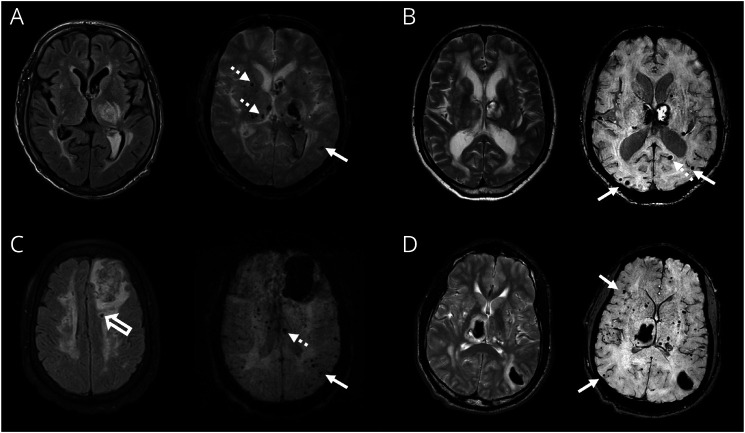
MRI Examples of Patients With Chronic Kidney Disease and Mixed Location Cerebral Small Vessel Disease (A) T2-weighted FLAIR and T2* GE MRI from a patient in their 40s with glomerulonephritis causing end-stage kidney disease requiring dialysis. There is a left thalamic ICH and mixed distribution microbleeds (lobar microbleeds, white arrows; deep microbleeds, dotted arrows). (B) T2-weighted and SWI MRI from a patient in their 60s with hypertension and stage 3a CKD (eGFR 49) with an acute left thalamic ICH, an old right thalamic hematoma, mixed pattern microbleeds, and severe WMHs. (C) T2-weighted FLAIR and GE MRI from a patient in their 40s with a history of hypertension, pre-eclampsia, and stage 3a CKD (eGFR 54) with albuminuria. There is a left frontal lobar ICH, a lacune in the left centrum semiovale (hollow arrow), severe WMH, and mixed distribution microbleeds. (D) T2-weighted and SWI MRI from a patient in their 30s with hypertension and stage 3a CKD (eGFR 56) with albuminuria. There are synchronous acute ICH in the right thalamus and left parietal lobe, moderately severe WMH, and florid mixed distribution microbleeds. CKD = chronic kidney disease; eGFR = estimated glomerular filtration rate; FLAIR = fluid attenuated inversion recovery; GE = gradient echo; ICH = intracerebral hemorrhage; SWI = susceptibility-weighted imaging; WMH = white matter hyperintensity.
